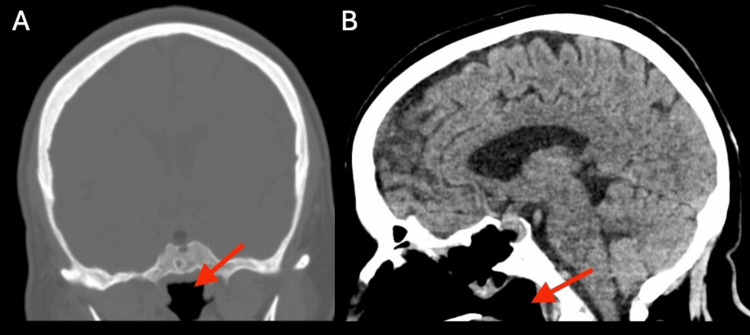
Coronal (A) and sagittal (B) non-contrast head CT performed 10 hours after transsphenoidal resection of the pituitary neuroendocrine tumor The red arrow indicates residual mass versus intrasellar hemorrhage measuring 2.2 cm × 1.3 cm × 1.6 cm.
